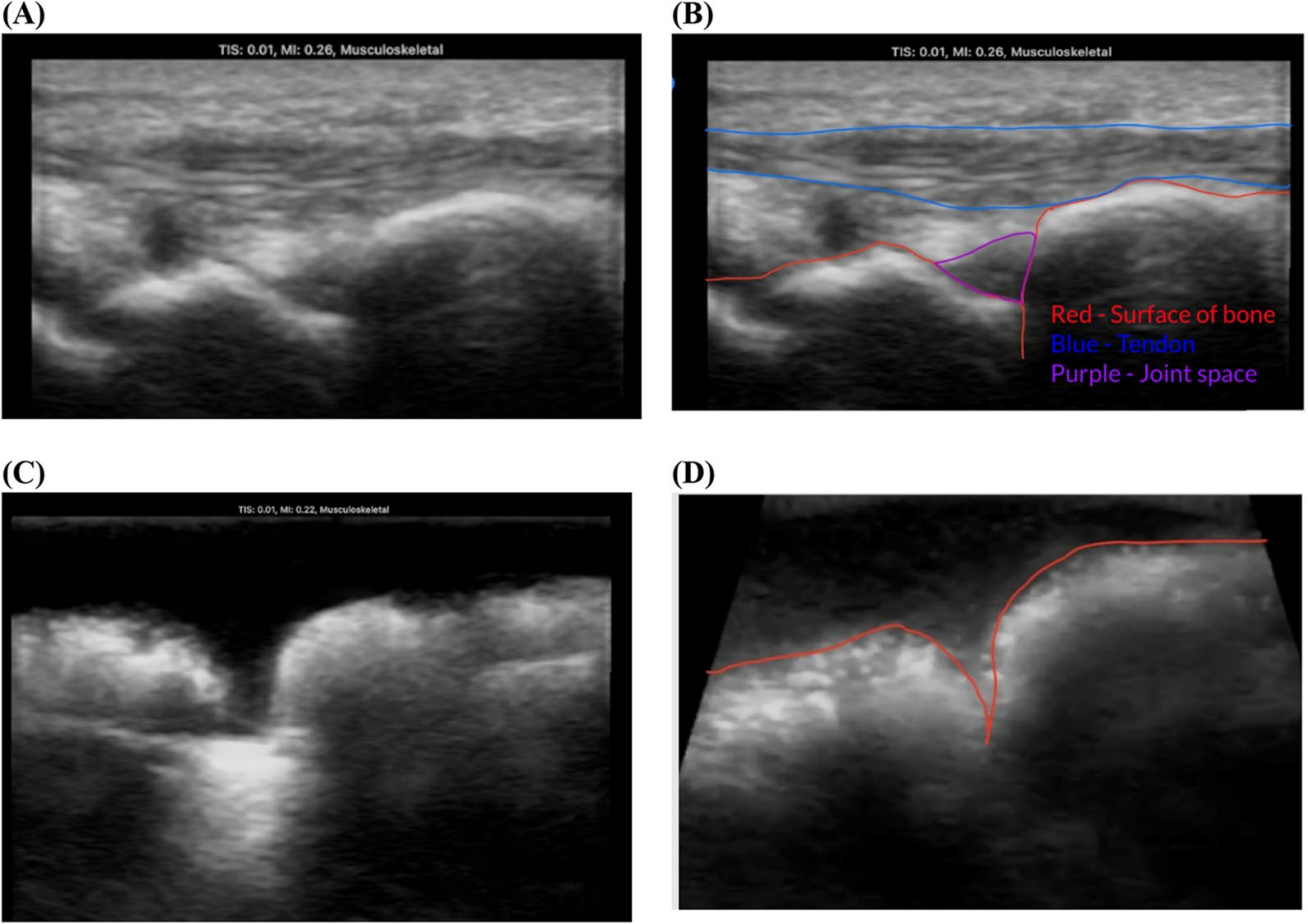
Comparison of ultrasound profiles between a human wrist and task trainer model. A Ultrasound profile of human wrist on longitudinal plane. Notable features in view, including the surface of the bone (red), a tendon (blue), and the joint space (purple) are outlined for reference. B Ultrasound profile of wrist task trainer on longitudinal plane. The surface of the 3D bone is outlined in red. Ultrasound images were captured using a Butterfly iQ + ultrasound probe on the Musculoskeletal setting
